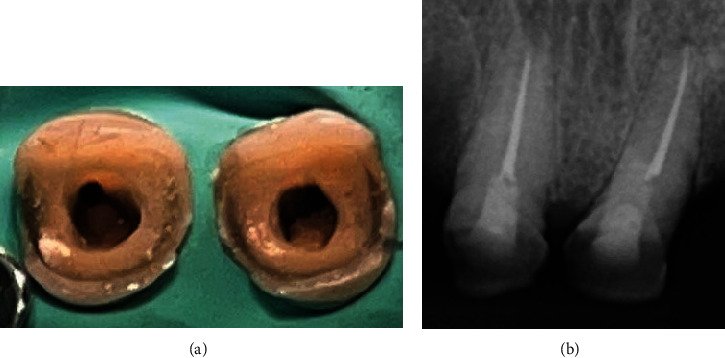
(a) Instrumentation of root canals completed; (b) final postoperative radiograph.
